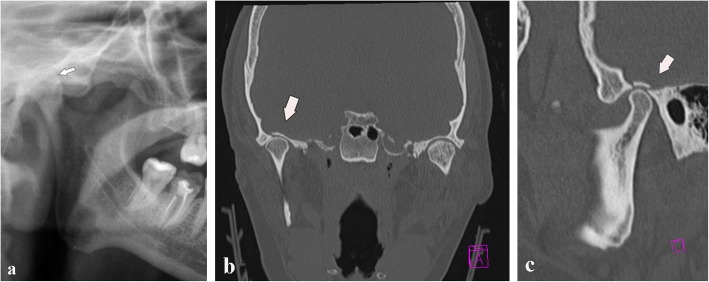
Fracture of the glenoid fossa of the temporal bone. a Cropped panoramic radiograph shows a reduction of the articular space. The condylar head seems to be higher than its usual location (arrow). b, c Computed tomography coronal and sagittal sections. A slight upward movement of the mandibular condyle associated with a glenoid fossa fracture of the temporal bone is observed (arrows)
